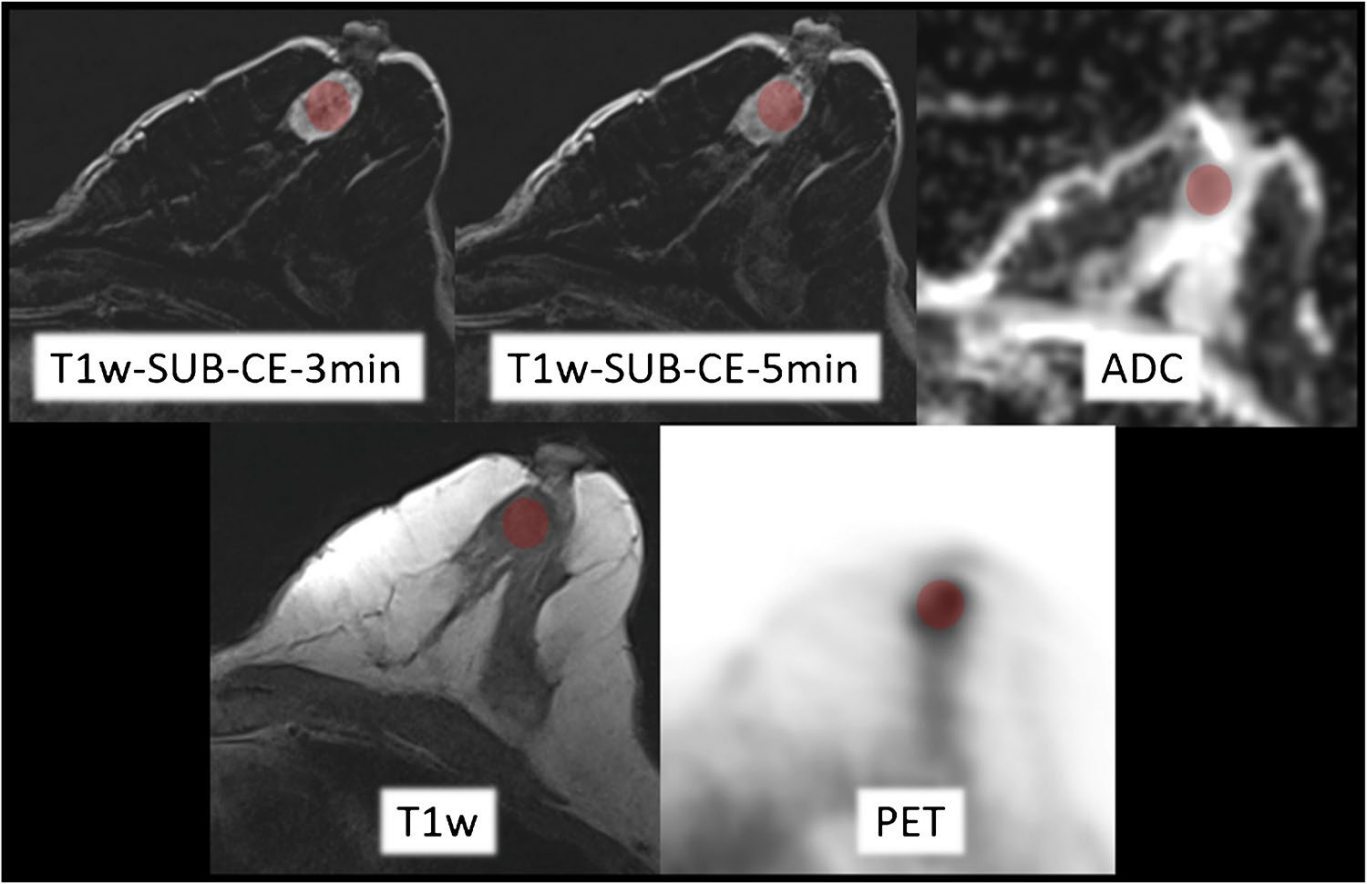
Example of the measuring procedure of a malign breast cancer lesion at different sequences of breast-[^18^F]FDG-PET/MRI dataset. The red circle represents the volume of interests (VOI)
